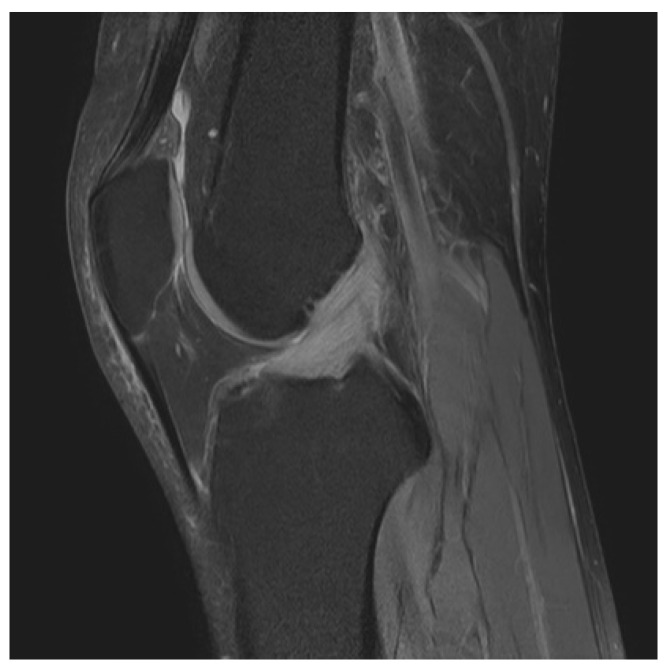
Preoperative proton density-weighted sagittal view of magnetic resonance imaging (MRI) shows overall high signal intensity and increased diameter of the anterior cruciate ligament (ACL) without discontinuity.
